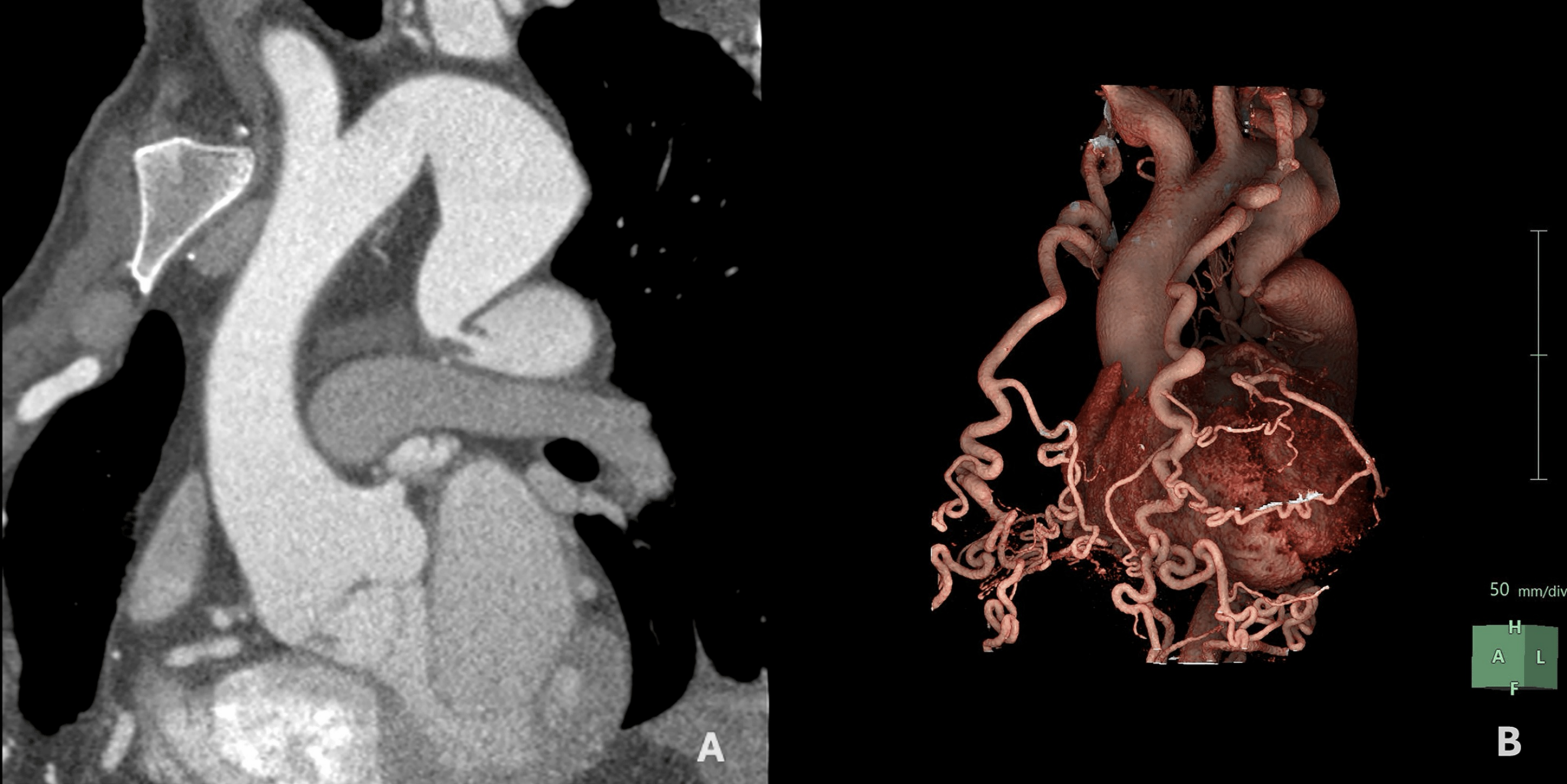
(A) Curved planar reformation of aortic CTA showing a severe postductal aortic coarctation with a minimal luminal diameter of 3 mm. (B) Volume-rendered reconstruction demonstrating marked dilation of both internal mammary arteries CTA: CT angiography
